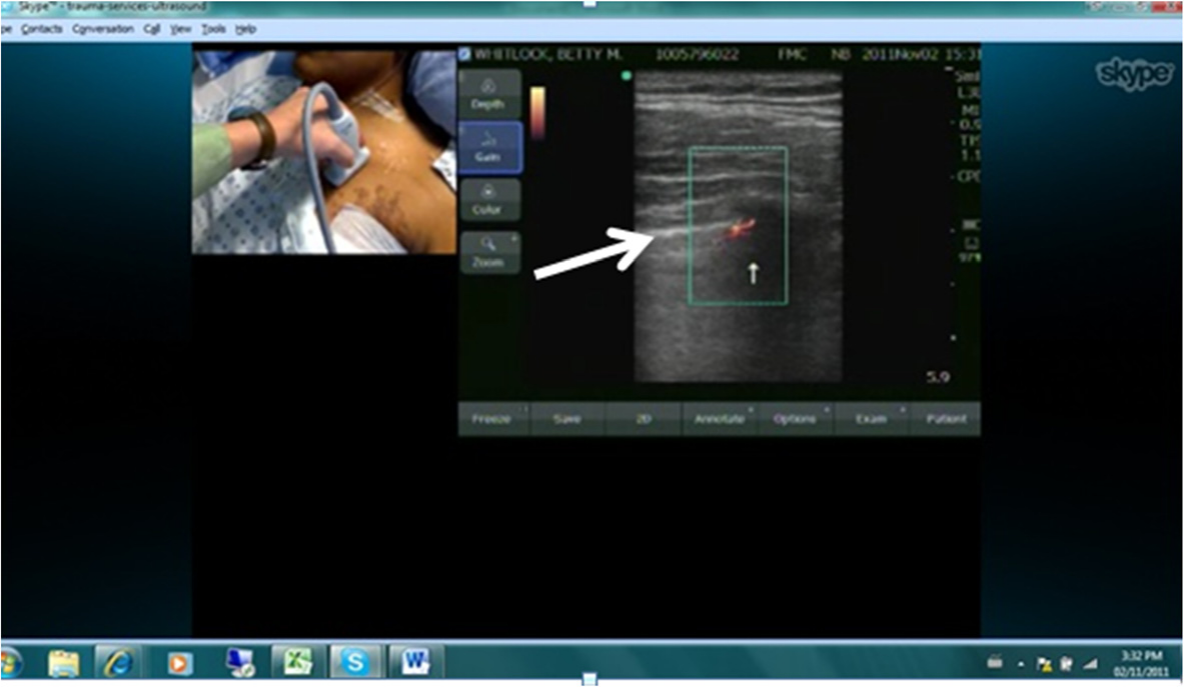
Case 1: remote mentor's computer screen. The screen demonstrated the nurse practitioner's placement of the ultrasound probe and the resultant ultrasound image that depicted a color power Doppler signal from the pleural interface, suggesting the presence of lung sliding at this anatomic location. The large white arrow designated the parietal-visceral pleural interface, and the small white arrow indicated the color power Doppler signal seen at this interface.
